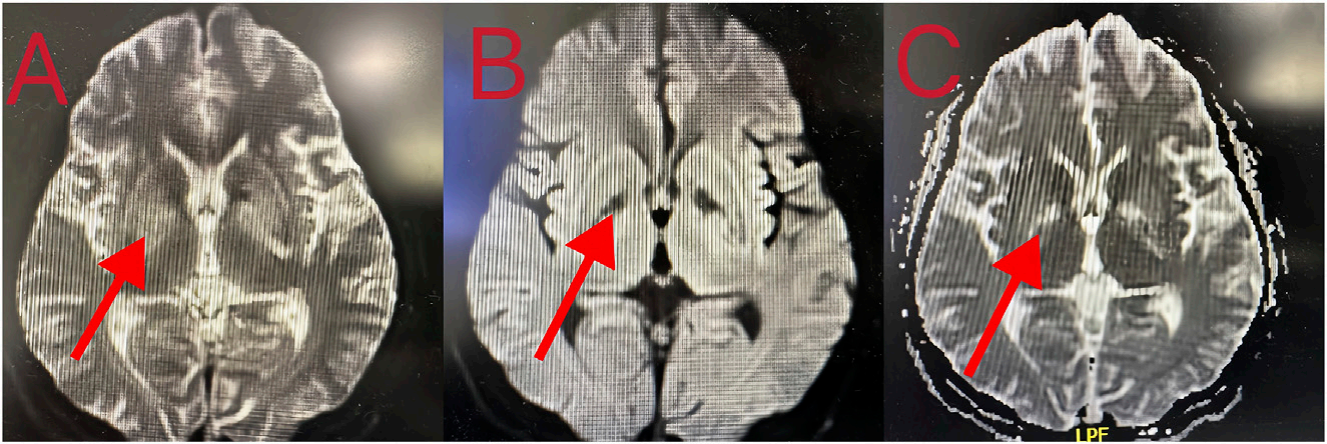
(A–C) Brain MRI showing that the density of nuclei in the bilateral basal ganglia was generally reduced, which was consistent with toxic encephalopathy.
